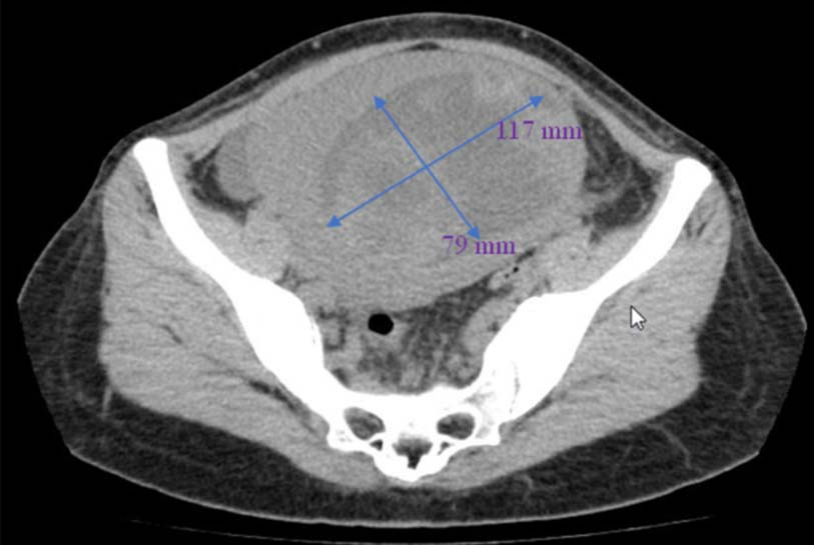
Computed tomography image of a heterogeneous structure (117×79 mm) in the uterus.
